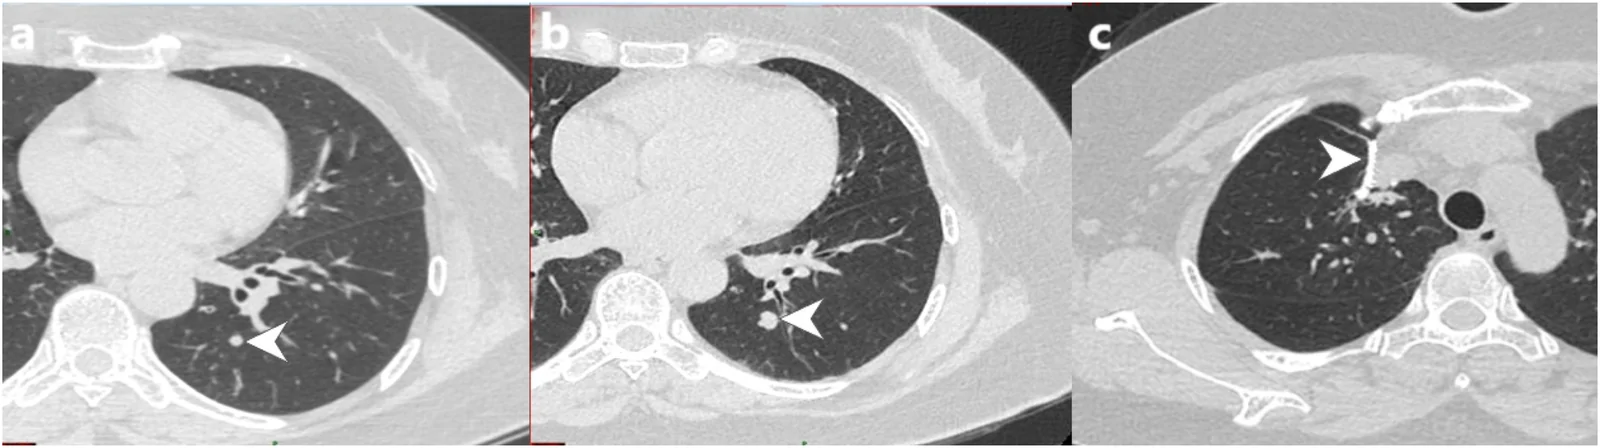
Pre-ablation axial thin-section chest CT (lung window). (a) Baseline scan obtained 6 months earlier shows a small, round solid nodule in the dorsal segment of the left lower lobe, with well-defined margins (arrowhead, 6.2 × 5.8 mm). (b,c) Follow-up 1 week before ablation: (b) the nodule (arrowhead, 10.4 × 7.2 mm) has clearly enlarged compared with baseline; (c) at the level of the apical segment of the right upper lobe, a linear high-attenuation staple line from the prior thoracoscopic segmentectomy is seen, with no evidence of local tumor recurrence at the surgical site.
